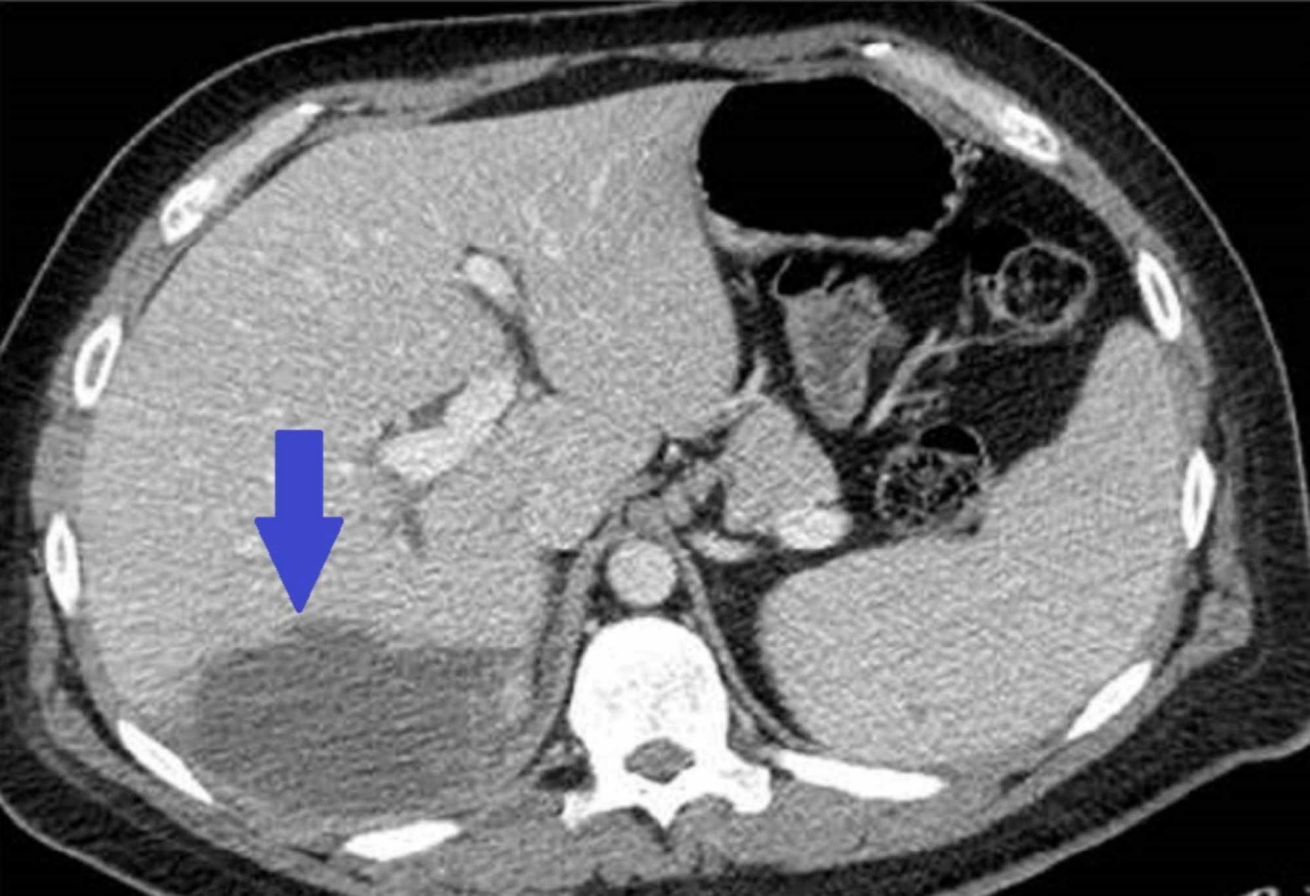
Abdominal computed tomography (CT) scan showing a rim enhancing subhepatic fluid collection
